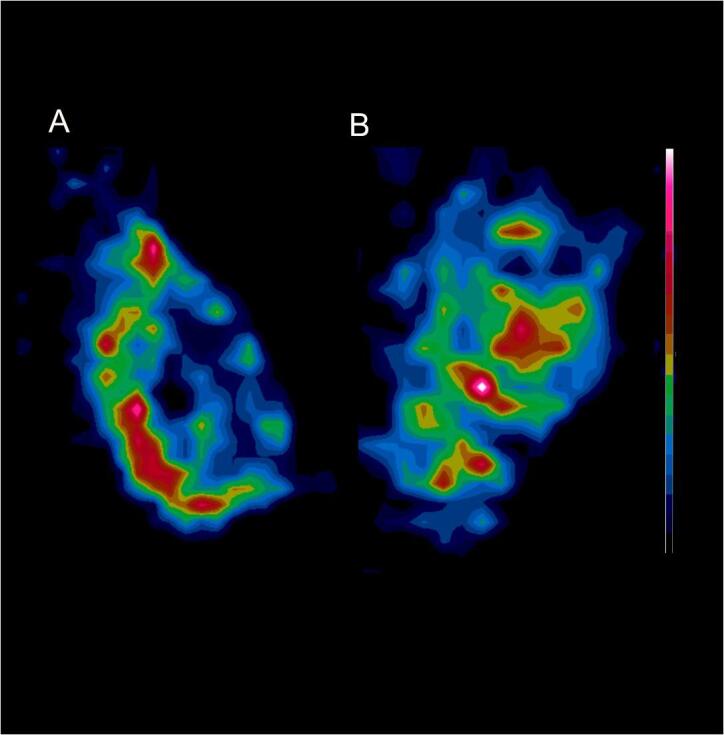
In vivo* SPECT images show cross sections of the 3D uptake of [^111^In]In-girentuximab-F(ab’)_2_. Image (A) is from a non-treated tumor of the FaDu model and image (B) is from a FaDu tumor treated with atovaquone.
